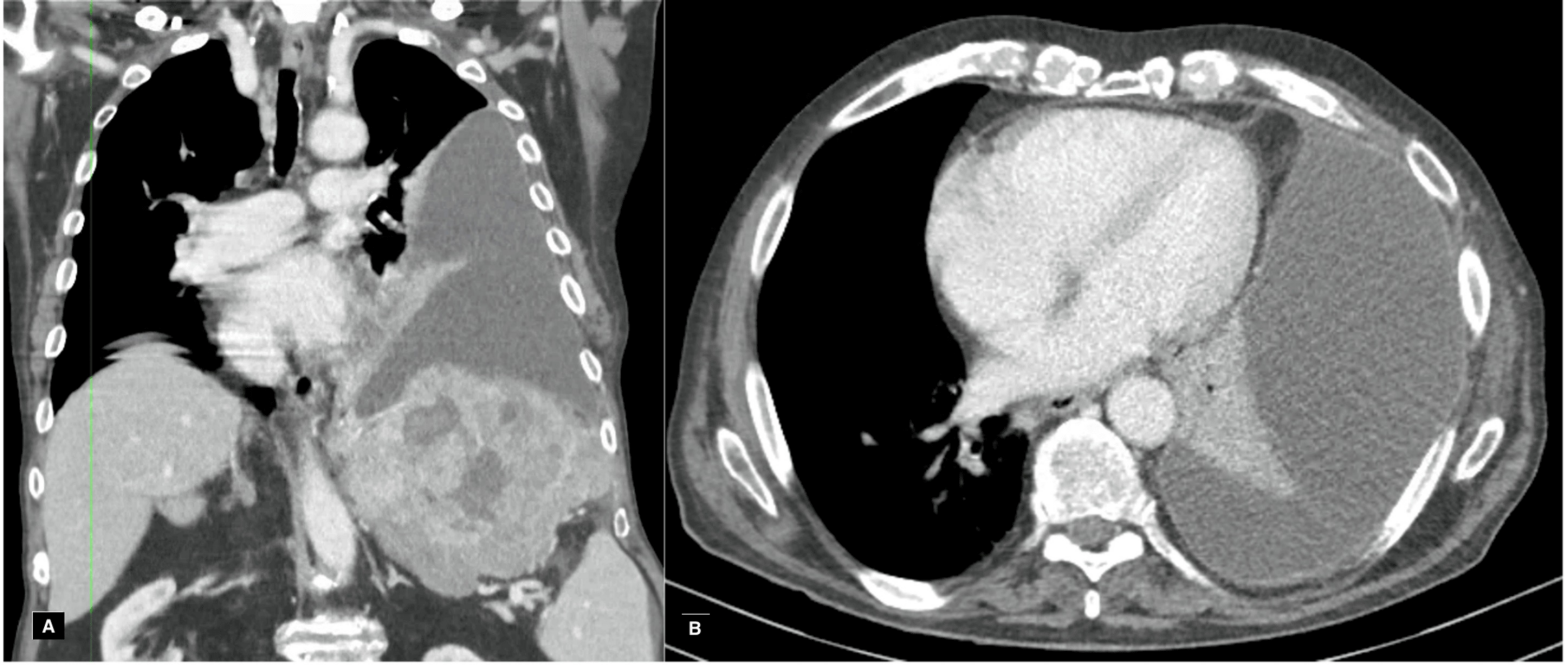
Chest and abdominal computed tomography showing pleural effusion in the left lung field, (A) coronal section and (B) axial section, with regular and diffuse pleural enhancement.
